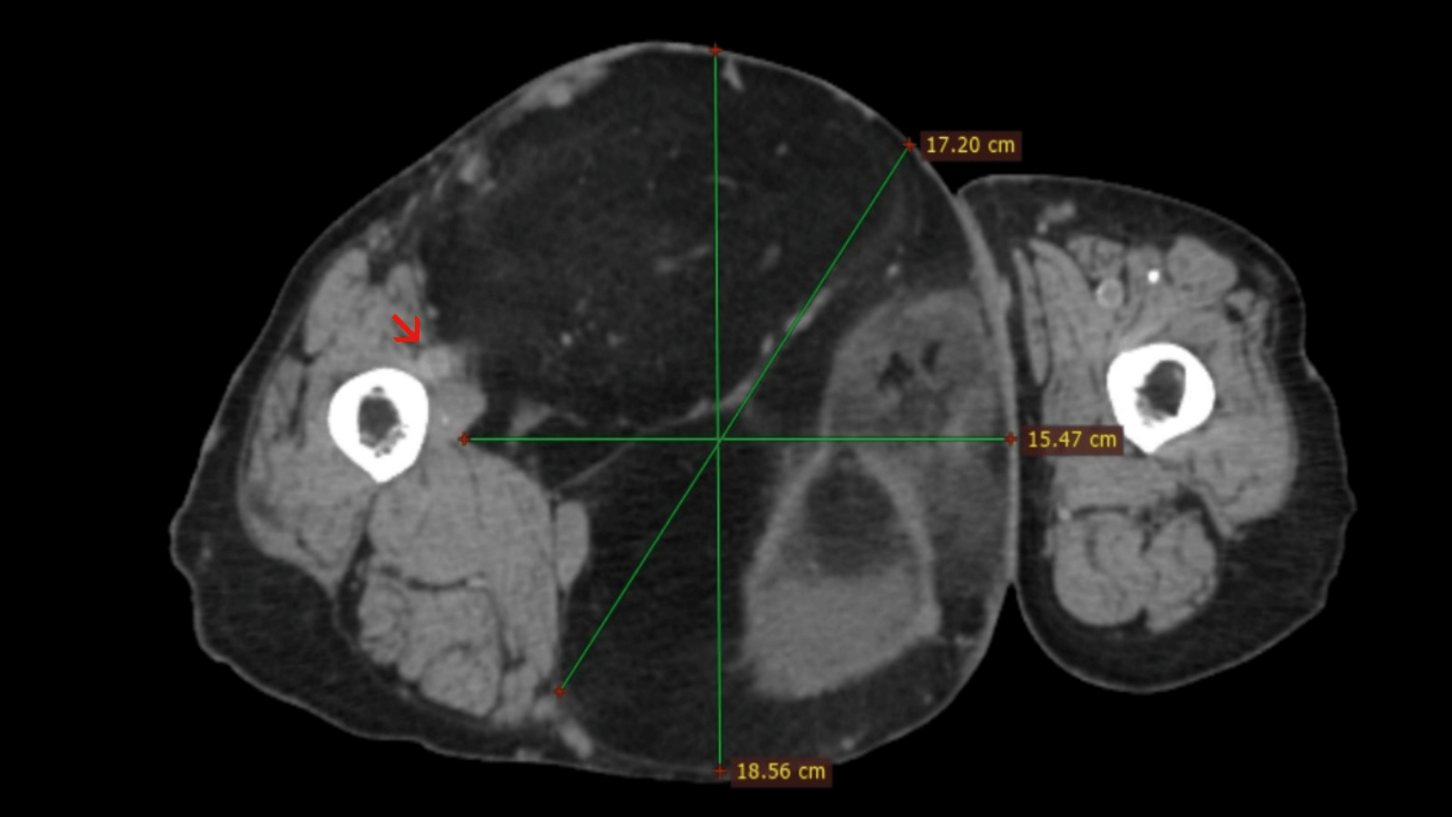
Axial CT image showing a giant solid mass in the right anterior thigh without involvement of vascular structures (right arrow).
